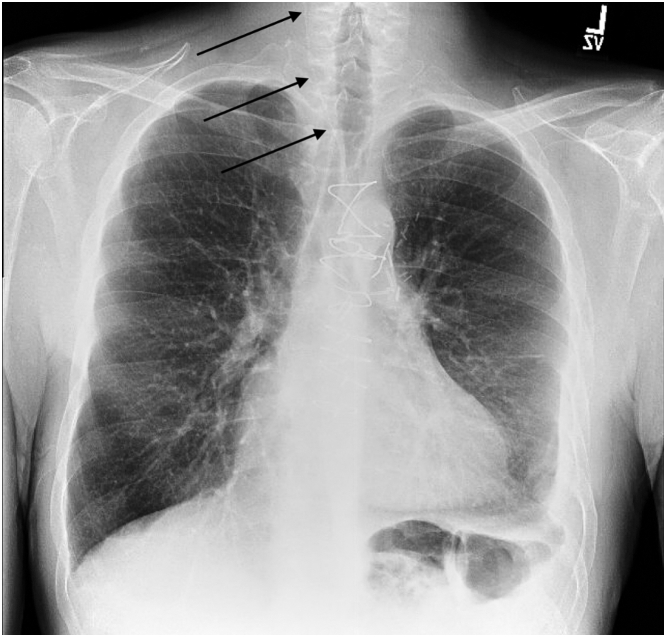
Preoperative Chest X-Ray Showing Migrated the TEPW in the Aorta and Carotid Artery Chest x-ray showing the retained temporary epicardial pacing wire tracing from the aorta, coursing along the aorta into the right common carotid artery indicated by the black arrows, taken during preoperative workup for wire retrieval.
